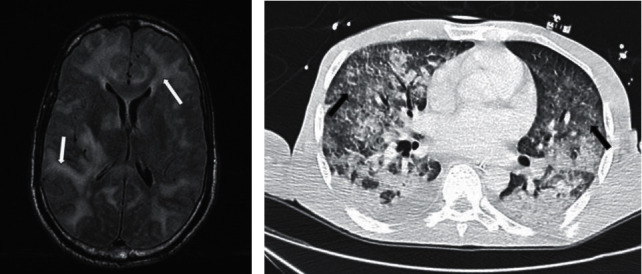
Imaging findings. (a) Cerebral MRI (axial, T2/FLAIR) showing cerebral edema compressing ventricles and gadolinium-enhancing T2/FLAIR white matter and basal ganglia hyperintensity, evoking acute necrotizing encephalopathy. (b) Chest CT scan showing bilateral ground-glass opacities.
